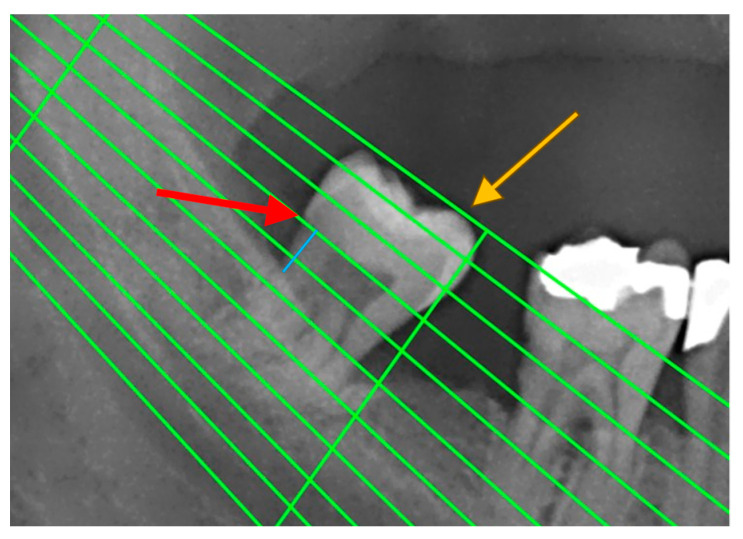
An example of measuring radiographic bone loss using a Schei ruler, where the red arrow indicates the line tangent to the cementoenamel junction, the yellow arrow indicates the line tangent to the highest point of the crown, and the blue line represents approximately 1.5 fields between the cementoenamel junction and the alveolar bone level.
